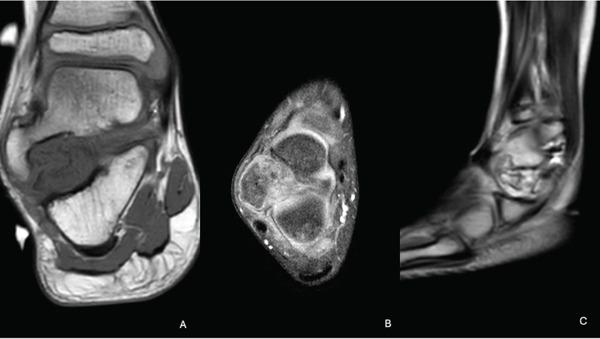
Coronal T1‐weighted (A), axial T1‐weighted (B), and sagittal T2‐weighted (C) MRI images demonstrating a cartilaginous outgrowth arising from the lateral talar body without epiphyseal communication.
